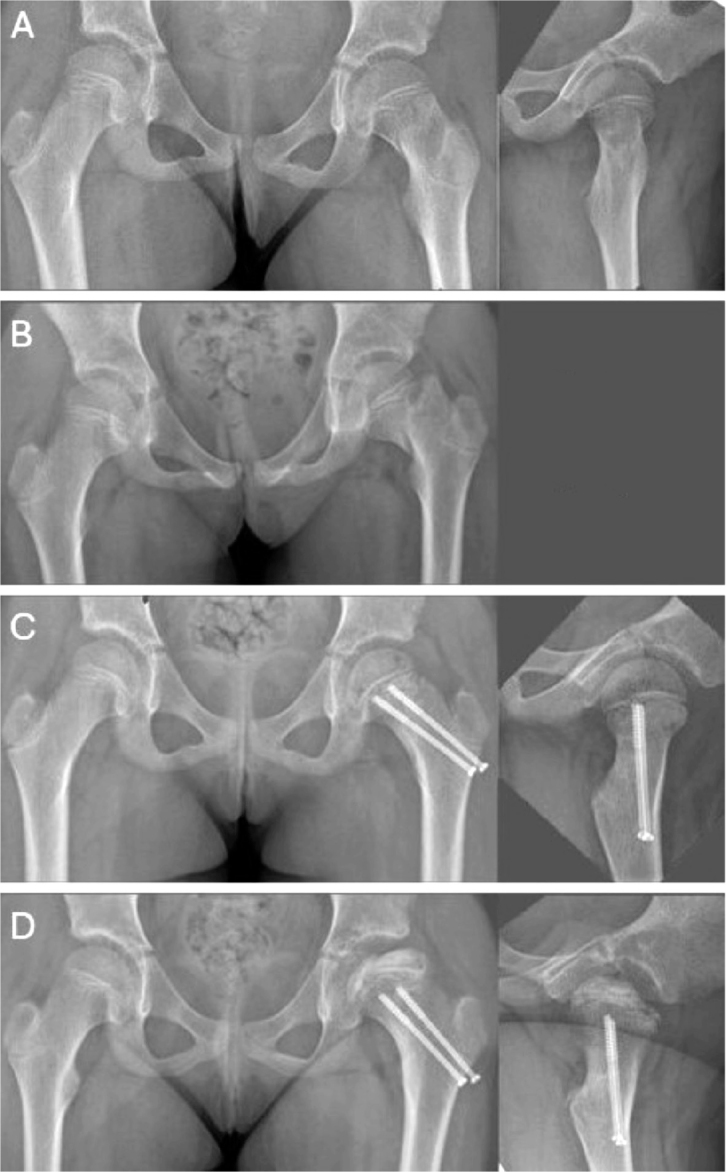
(A) Primary radiographs showing a non-dislocated Delbet II fracture in an 8-year-old girl. (B) Loss of alignment at 10 days’ follow-up. (C) Ratliff type 1 AVN at 3 months post-injury. (D) AVN at 11 months post-injury.
